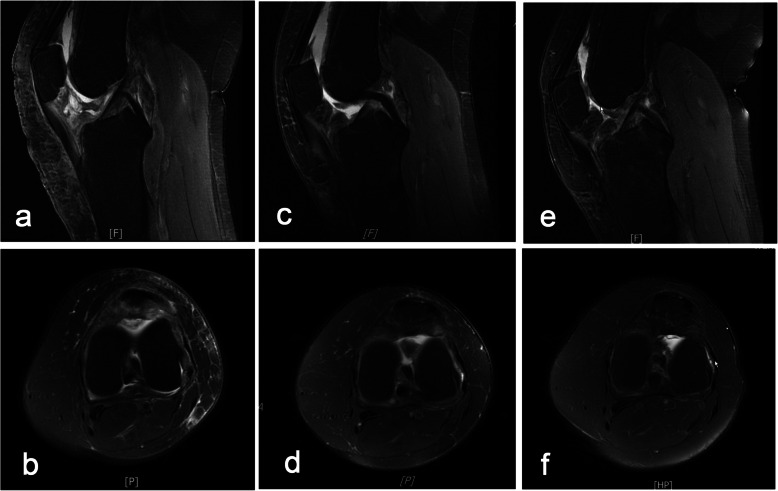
MRI scan at the follow-up. a, b No recurrence was observed on either the sagittal or axial MRI scans at the 1-month follow-up. c-f No recurrence was observed at the 6- or 18-month follow-up
